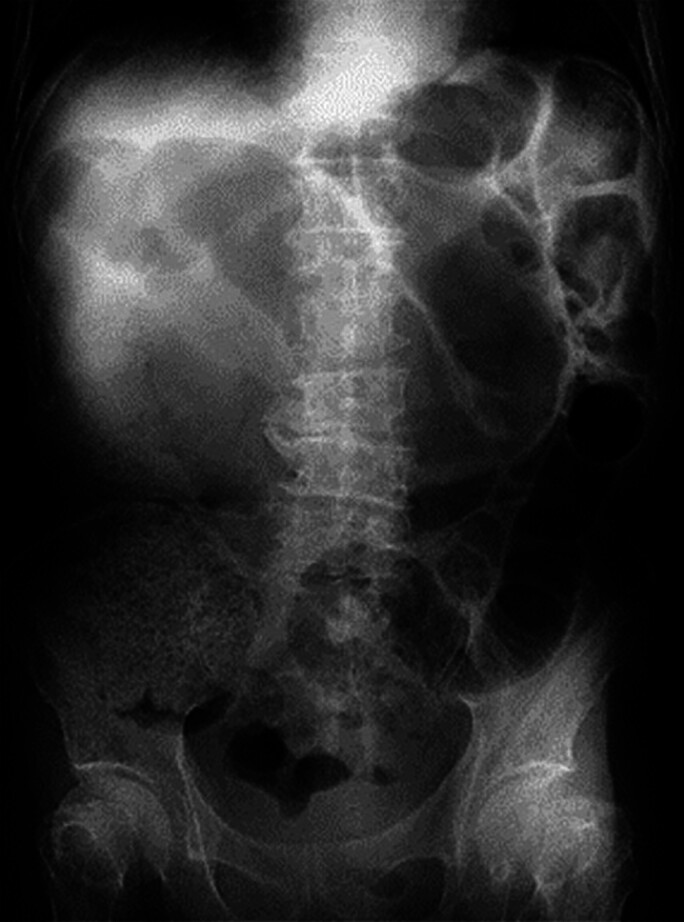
Abdominal radiographs in an 80-year-old man with bowel obstruction. Intestinal dilatation and niveau were observed.
